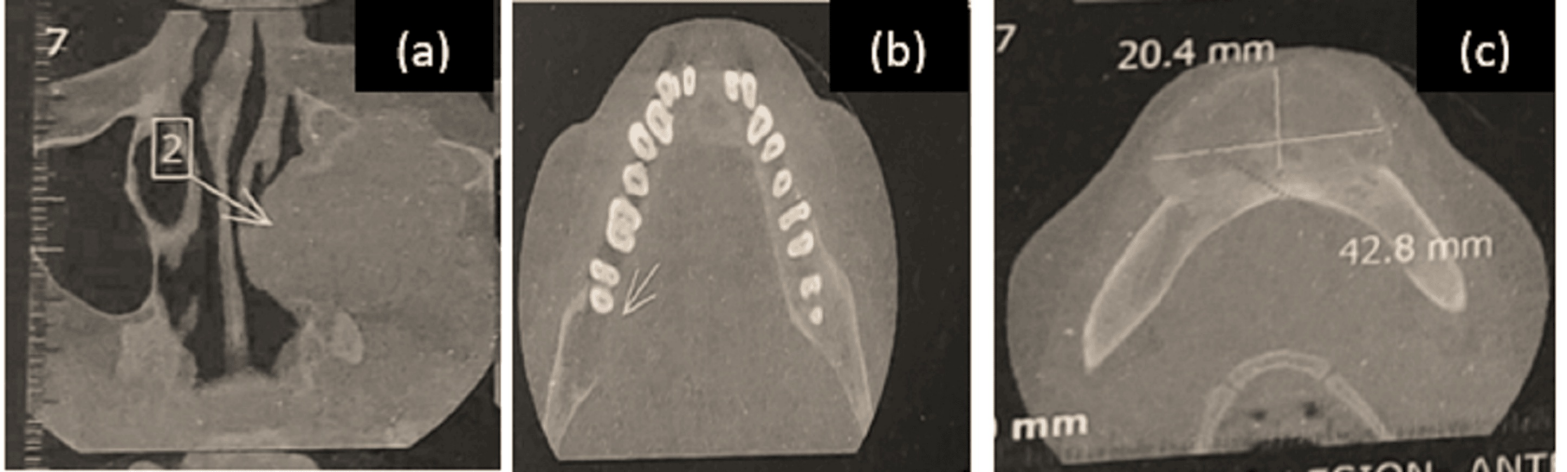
Cone beam computed tomography (CBCT) Cone beam computed tomography (CBCT) imaging showing (a) axial section revealing heterogenous radio-opaque to radiolucent expansile lesion involving the left maxillary sinus, (b) axial section revealing a radiolucency with non-corticated borders in the right posterior mandibular region, with a breach in the lingual cortex, and (c) axial section revealing a heterogenous radiopaque to radiolucent expansile lesion in the mandibular symphysis.
